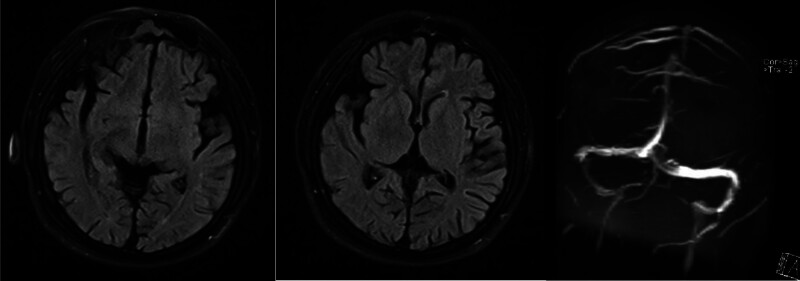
2022 MRI + MRV: mild cerebral atrophy; the blood flow signals in the right sigmoid sinus and internal jugular vein lumen were slightly weaker, with a possible localized narrowing at the terminus of the sigmoid sinus. The enhancement of the superior sagittal sinus lumen was locally diminished, showing significant improvement. MRI = magnetic resonance imaging, MRV = magnetic resonance venous imaging.
